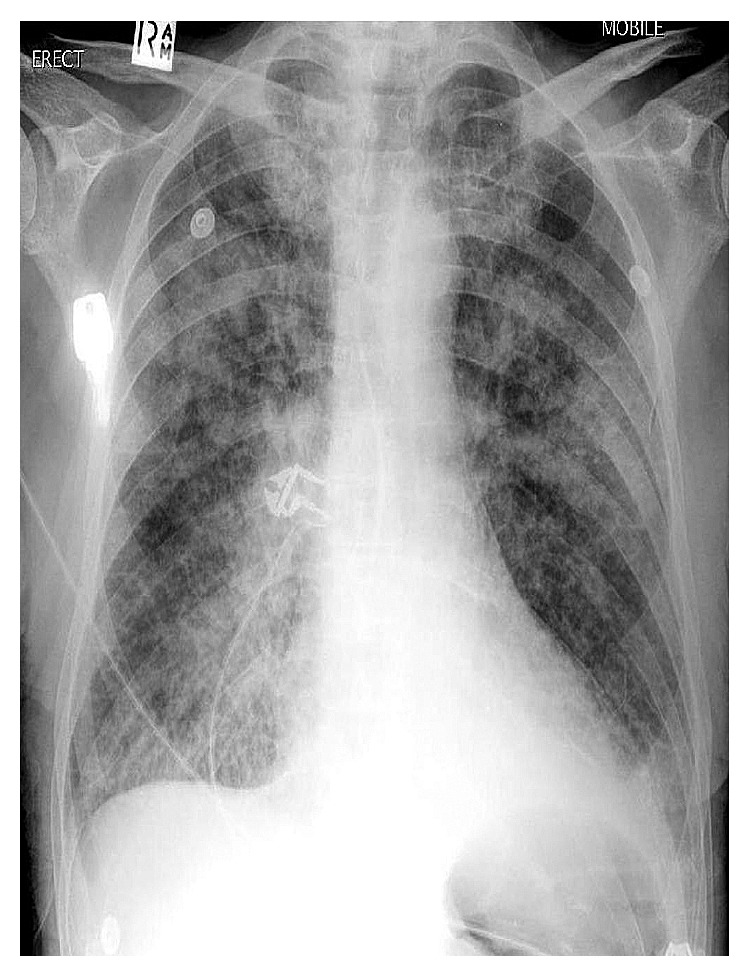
Chest X-ray of patient with SHS showing bilateral extensive pneumonic consolidation.
